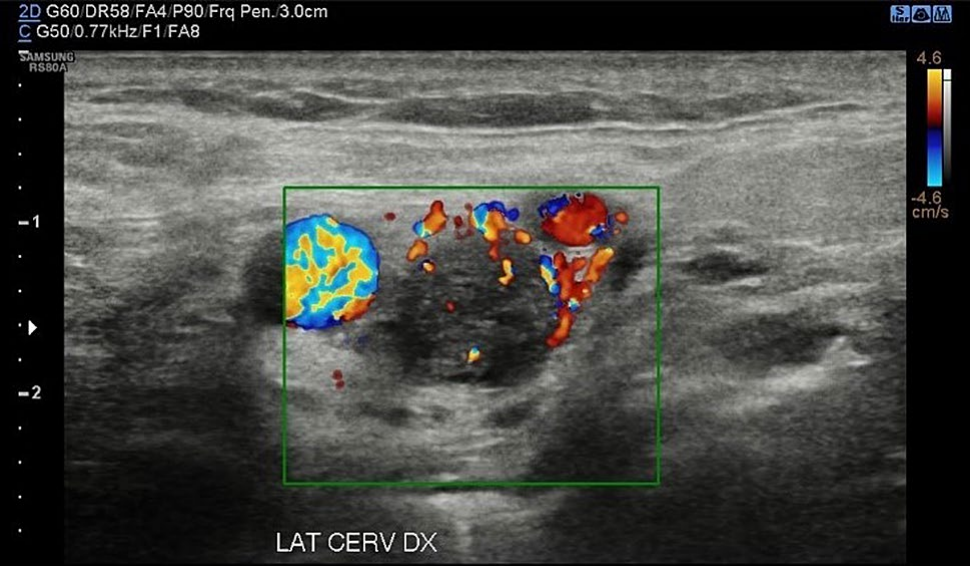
A predominantly peripheral vascularization with some centripetal vessels at Color-doppler
